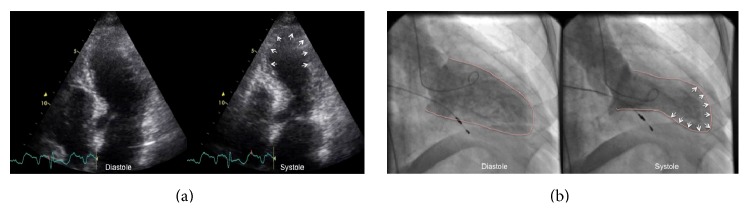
(a) Transthoracic echocardiography (apical four-chamber view) during the initial admission, demonstrating apical ballooning (white arrows). (b) Left ventriculography images in diastole and systole, showing typical type of takotsubo cardiomyopathy with apical ballooning, dyskinesia (white arrows), and left ventricle basal hypercontractility.
